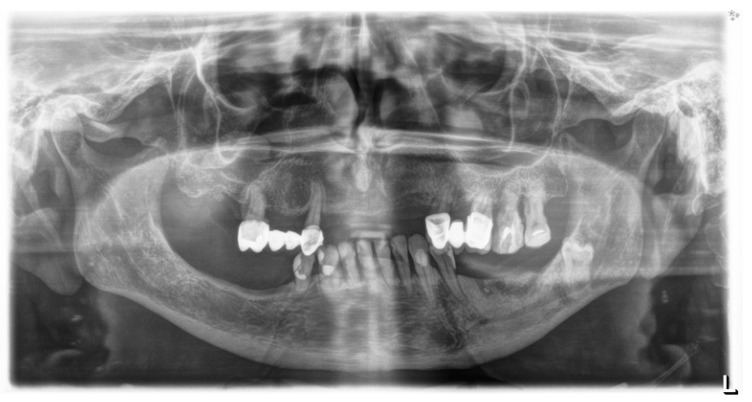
X-ray of the patient before treatment. Bone necrosis visible on the left side of the mandible.
